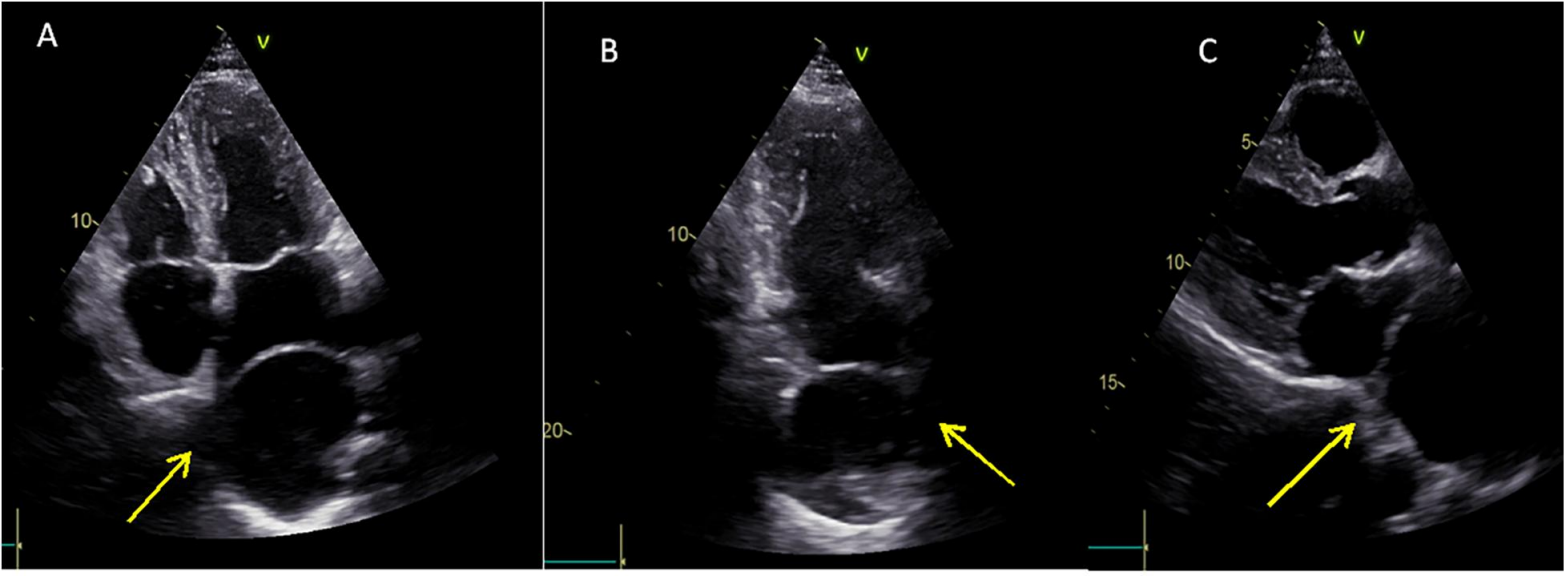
Transthoracic echocardiography—four-chamber view (A), two-chamber view (B), and parasternal long-axis view (C) showing an extra cardiac echolucent cavity that compresses the left atrium (yellow arrows).
